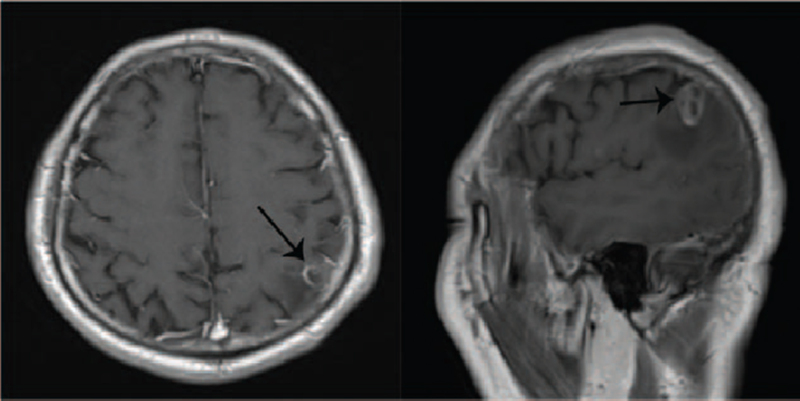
Brain MRI suggested cerebral metastases (October 2020). MRI = magnetic resonance imaging.
